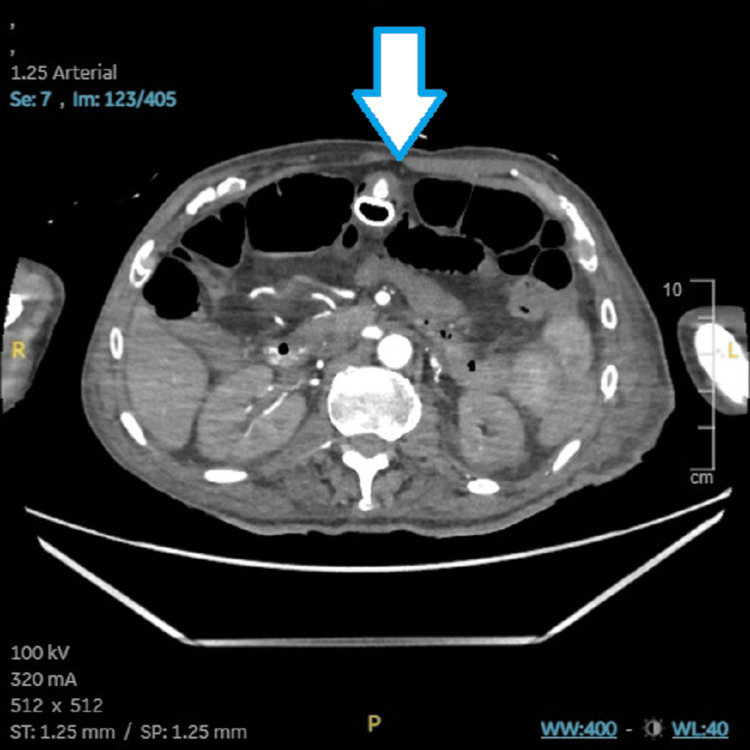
Contrast-enhanced axial CT of the abdomen showing markedly dilated, fluid-filled small bowel loops proximal to the obstructive transition point in the ileum, located immediately adjacent to the PEG insertion site. PEG, percutaneous endoscopic gastrostomy
